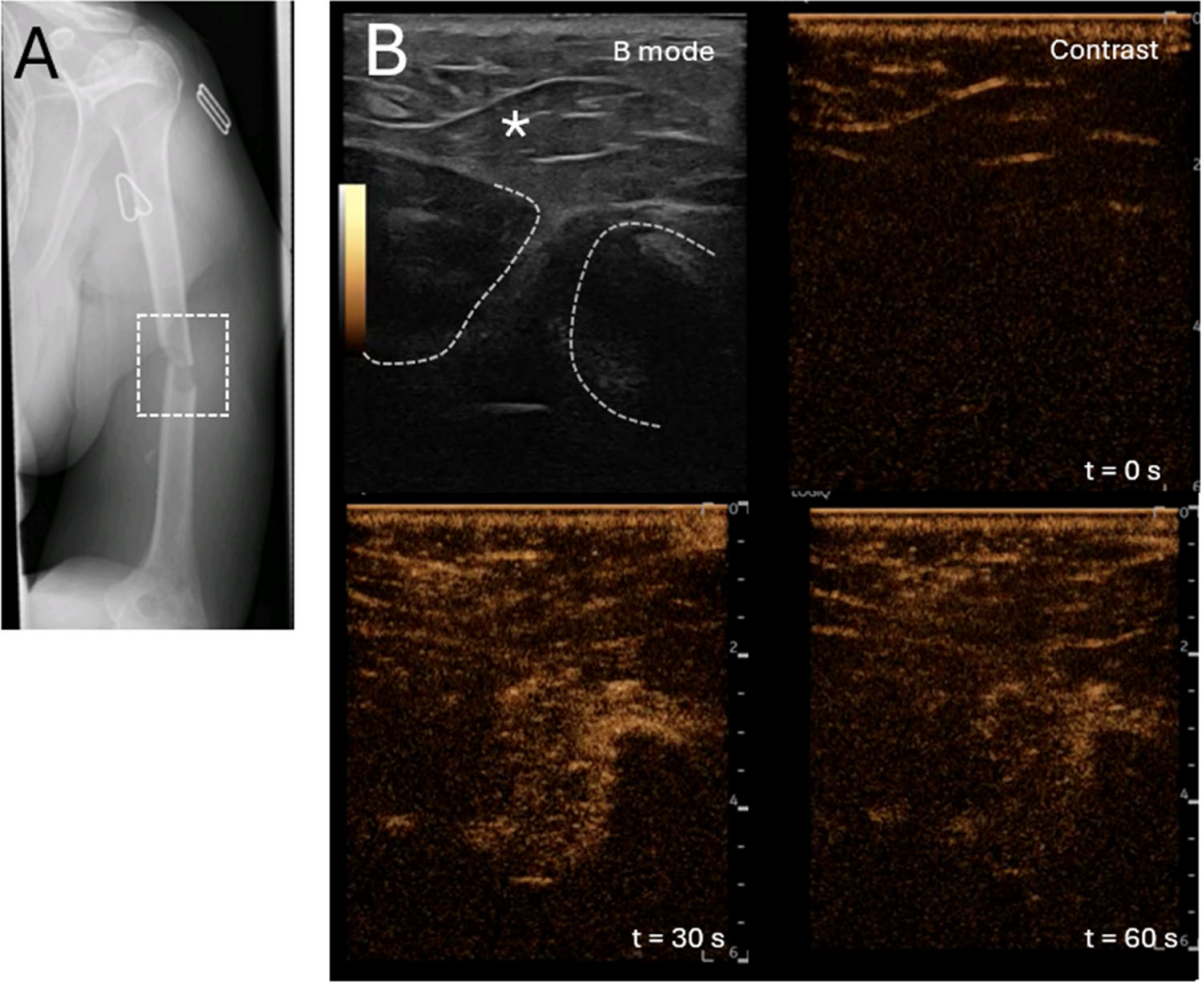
Contrast agents can be detected in humeral fracture gaps. (A) Patients were recruited with displaced fractures of the humerus as identified on radiographs. (B) The fracture gap was identified as a discontinuity in the bone, as shown in the B mode image with the bone edges indicated by dotted white lines and superficial muscle by *. Contrast perfusion of the fracture gap was evident as an increase in contrast over a period of 100 s of seconds post perfusion.
